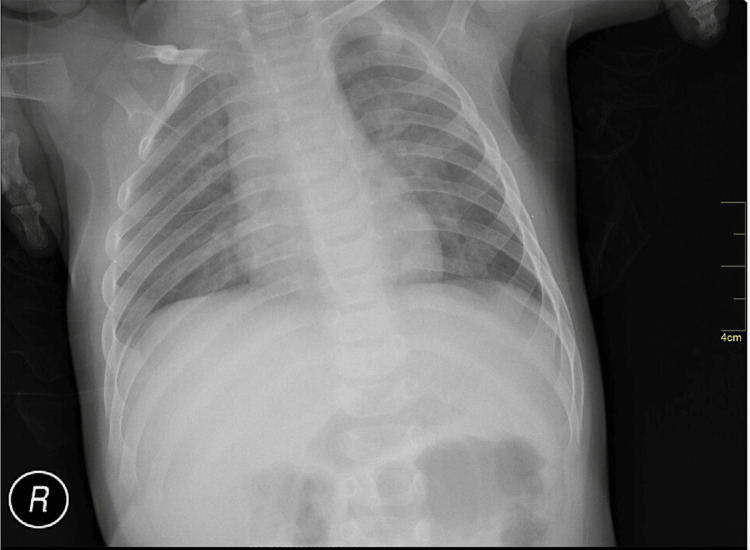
Normal Chest Radiograph Source: Nevit Dilmen. Available at: https://commons.wikimedia.org/wiki/File:Medical_X-Ray_imaging_MLK05_nevit.jpg. Accessed May 22, 2024. Licensed under CC BY-SA 3.0 (https://creativecommons.org/licenses/by-sa/3.0/).
